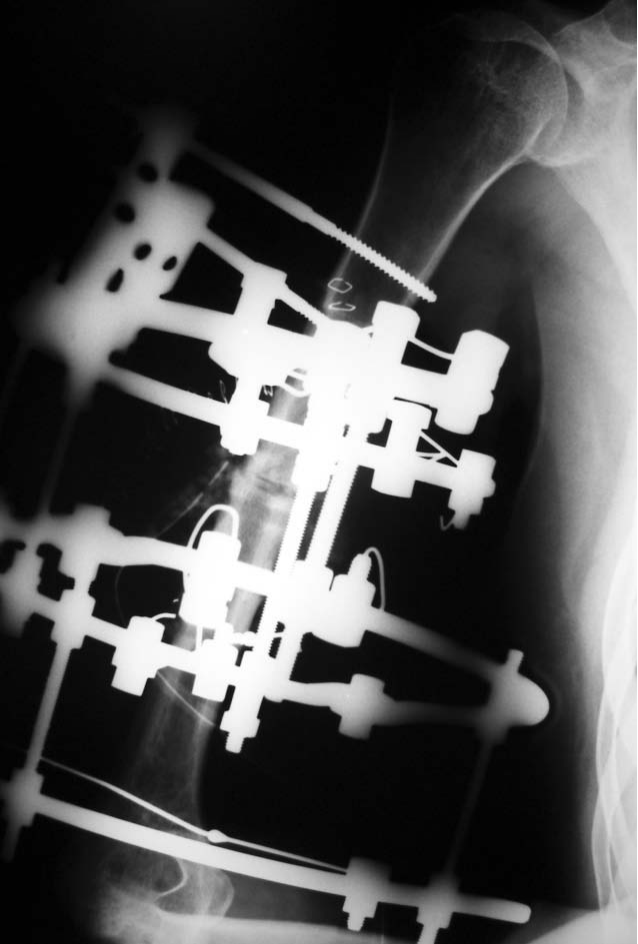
Radiograph of the3-ring frame.
